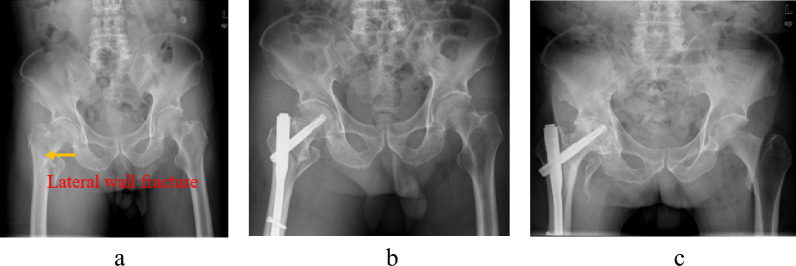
A 72-year-old female was treated for a right intertrochanteric fracture with lateral wall fracture (AO type A2.2) via closed reduction and internal fixation. a Radiograph of right hip showing intertrochanteric fracture with lateral wall fracture. b immediate postoperative radiograph after intramedullary nail. c Five years follow-up radiograph showing bone union and hip joint arthritis
